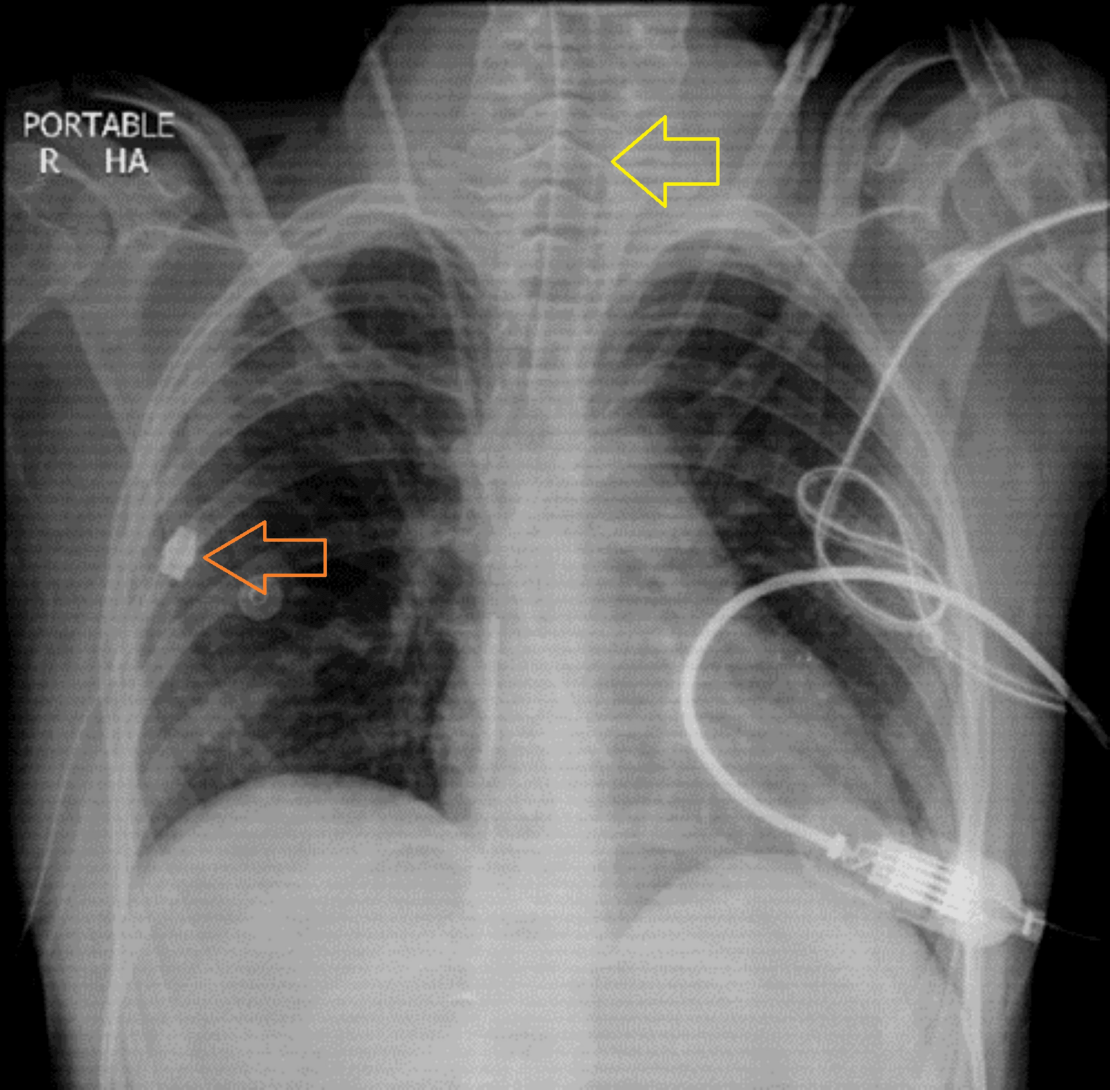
Chest X-ray taken on the ninth day of hospitalization, after clamping the chest tube for 24 hours, with the chest tube (orange arrow) and endotracheal tube (yellow arrow) in place
